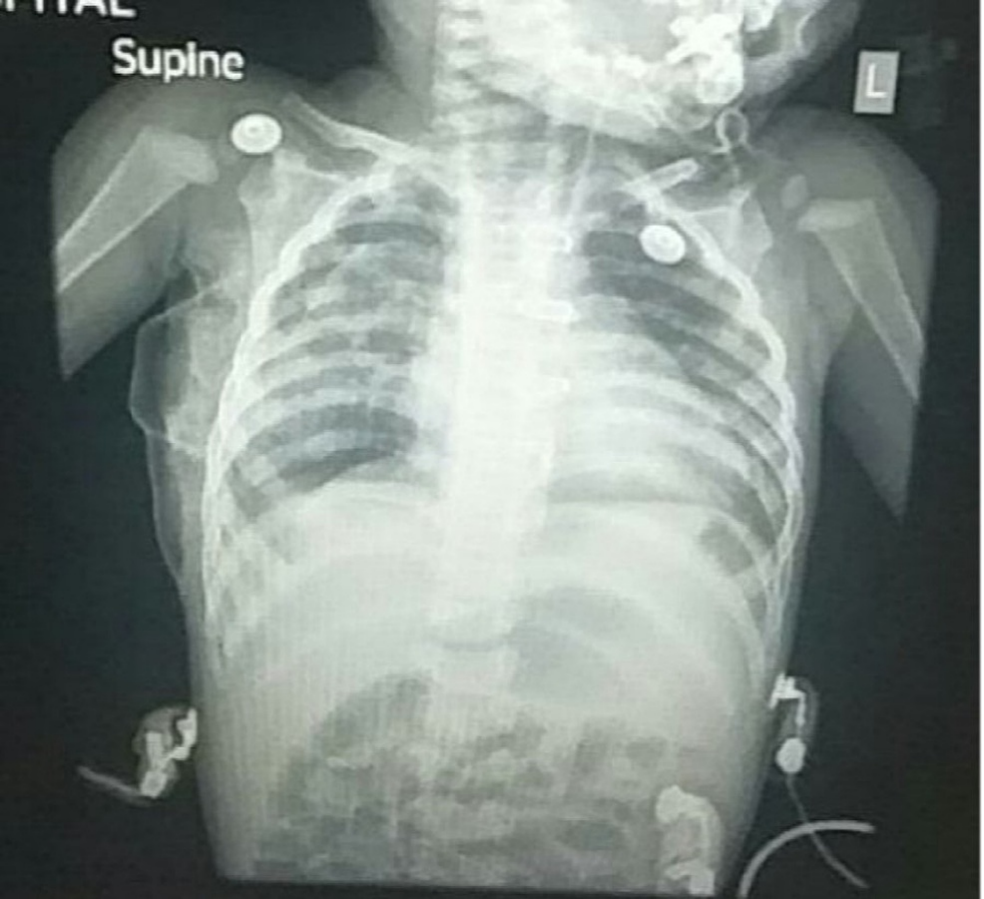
Postoperative chest X-ray showing good expansion of the right lung
